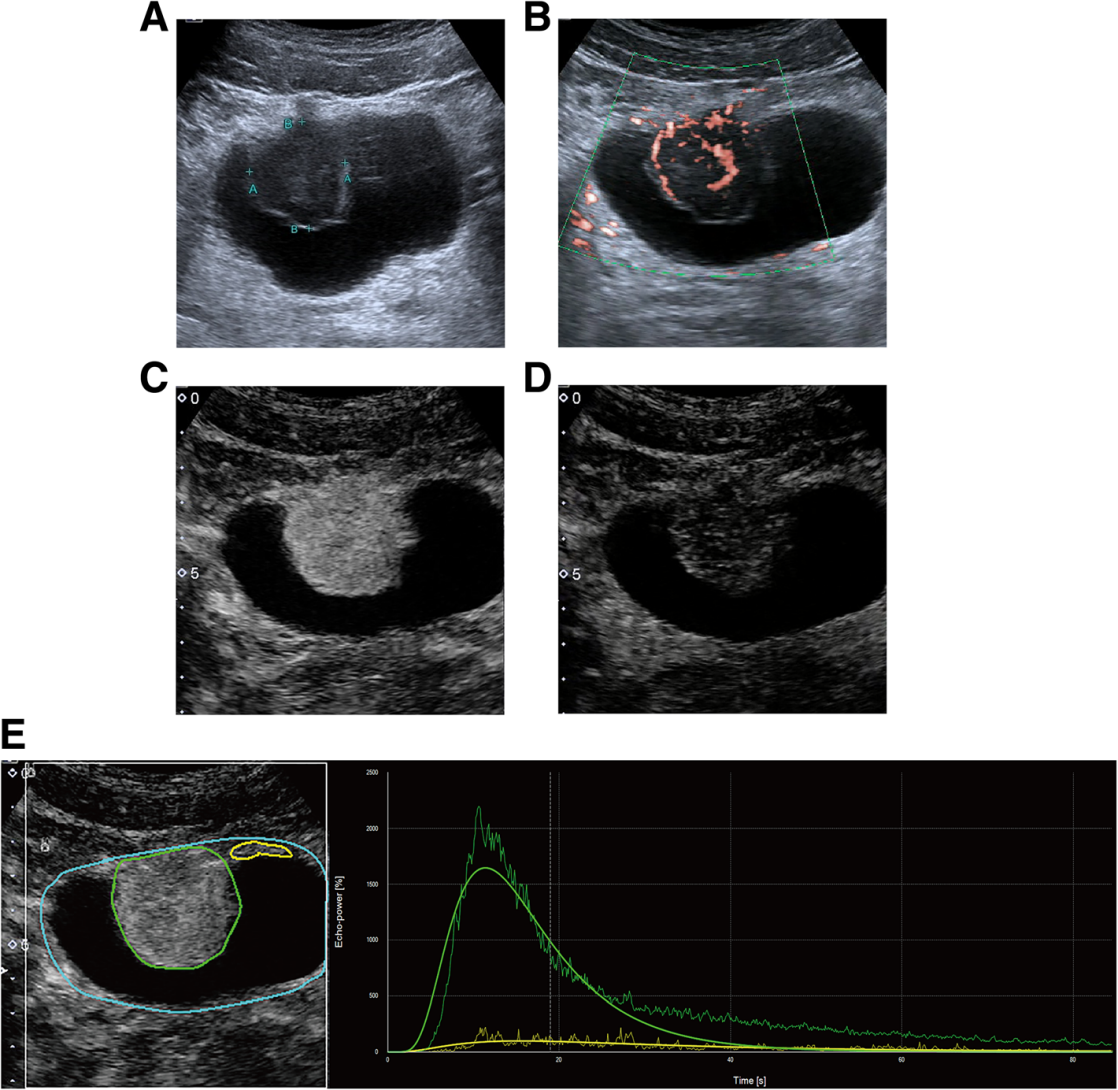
Ultrasonography and contrast-enhanced ultrasound (CEUS) imaging of large cell neuroendocrine carcinoma (LCNEC) in the urinary bladder. a Sonography showed a hypoechoic mass with hyperechoic margins in the anterior wall of the urinary bladder. b Superb microvascular imaging (SMI) showed strong blood flow signals in the mass. c CEUS imaging showed that the lesion achieved hyper-enhancement in the early phase in comparison to the bladder wall. d The lesion showed hypo-enhancement compared to the bladder wall. e Time-intensity curves (TICs) was created by analysing the ROI (green) positioned in the tumor and the reference ROI (yellow) in the bladder wall. TICs showed the lesion was initially enhanced at 9 s, the time to peak was 13 s and wash-out occurred at 40s
